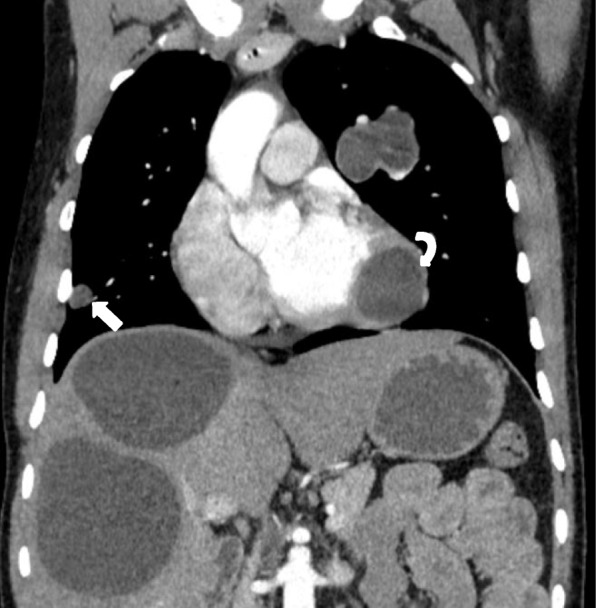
Contrast enhanced chest and upper abdominal CT coronal section: In addition the previously seen lesions in left lung and liver, a small pleural based cystic lesion in the right lung (arrow) as well as a larger lesion in the cardiac apex are seen (Curved arrow)
